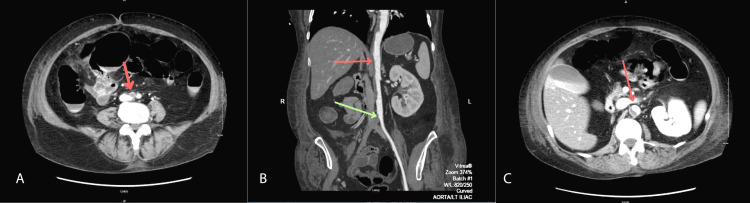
CT angiogram of aorta with run-off using intravenous contrast A: Axial view of right common iliac artery occlusion immediately distal to aortic bifurcation (red arrow); B: Coronal view of aortic plaque (red arrow) and common iliac artery occlusion (green arrow); C: Axial view of aortic plaque (red arrow)
